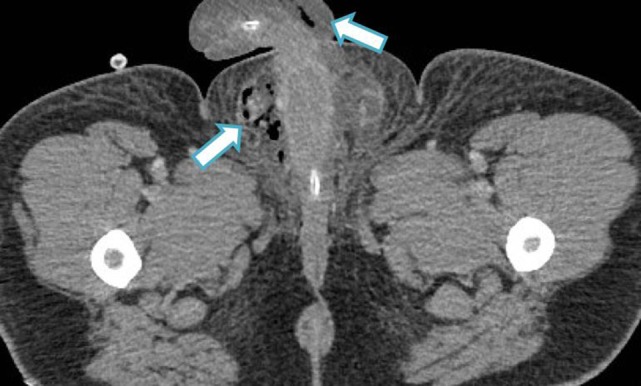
CT image (axial slice) showing gas within the scrotum and penile shaft.
